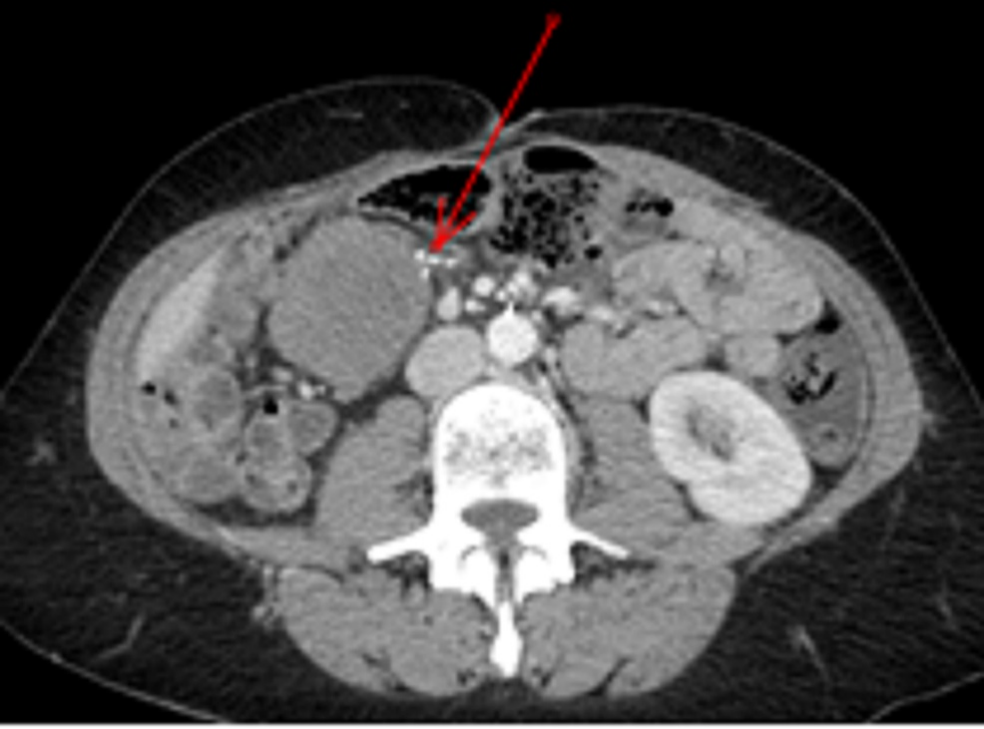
Case 1. Contrast-enhanced abdominal CT scan This shows a well-defined homogenous non-adipocytic round mass in the mesentery close to the staple line of the ileocolic anastomosis (red arrow).
